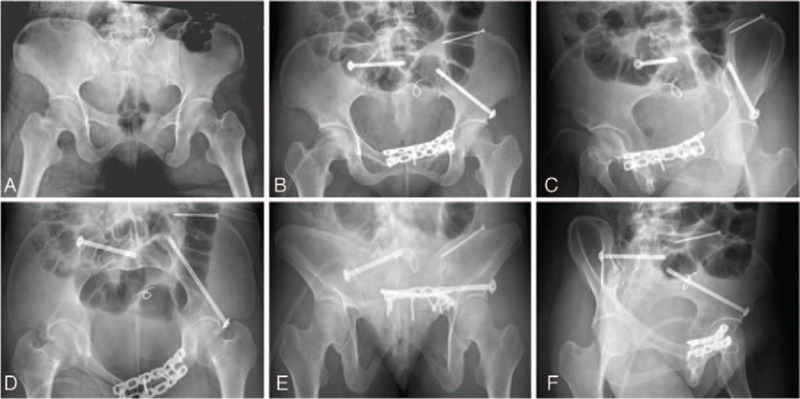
(A) Preoperative radiograph showed that there was bone discontinuity and displacement in the left superior and inferior pubic ramus, and a gap was showed though the ilium. (B–F) Postoperative radiographs demonstrated that the positions of PPSs were excellent. PPSs = percutaneous pelvic screws.
